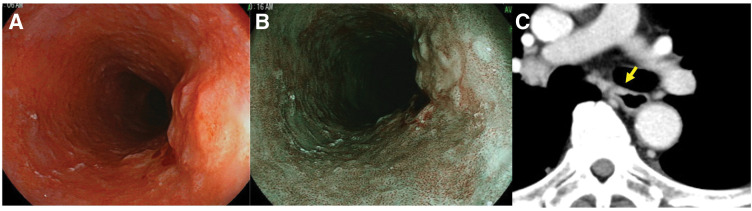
Images of esophagogastroscopy (A: white light; B: narrow band imaging) and chest CT scans (C) show a 0–IIc+0–Is lesion mainly occupying the right wall of the middle thoracic esophagus. The yellow arrows indicate the area of wall thickening on CT (C).
